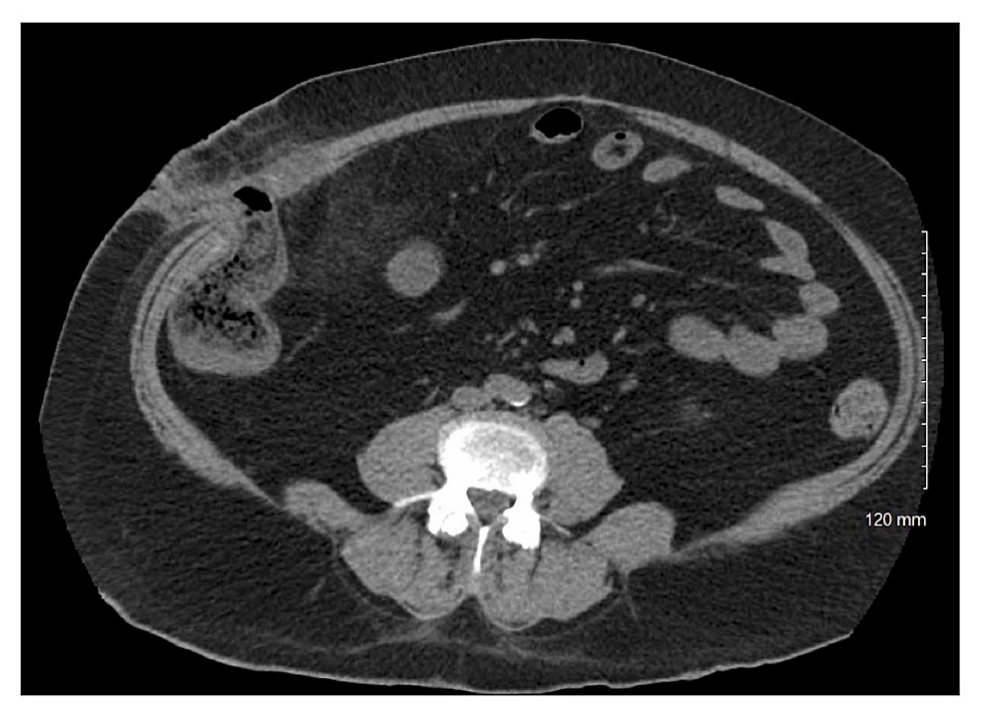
CT abdomen post-cecostomy showing improvement in colonic distention (120 mm ruler for scale).
